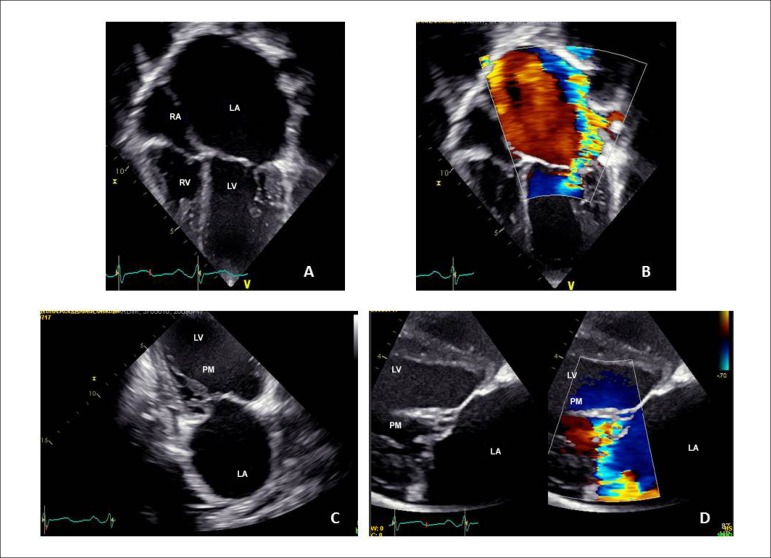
Echocardiogram showing the marked enlargement of the left heart
